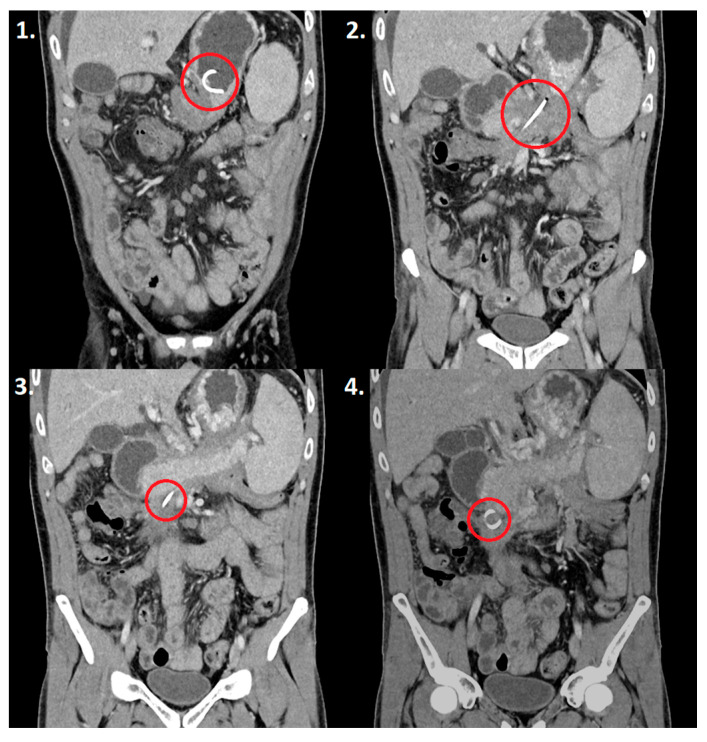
CT scan showing a single double-pigtail stent during one of the patient follow-ups 6 months after the procedure (in the red circle images 1–4).
